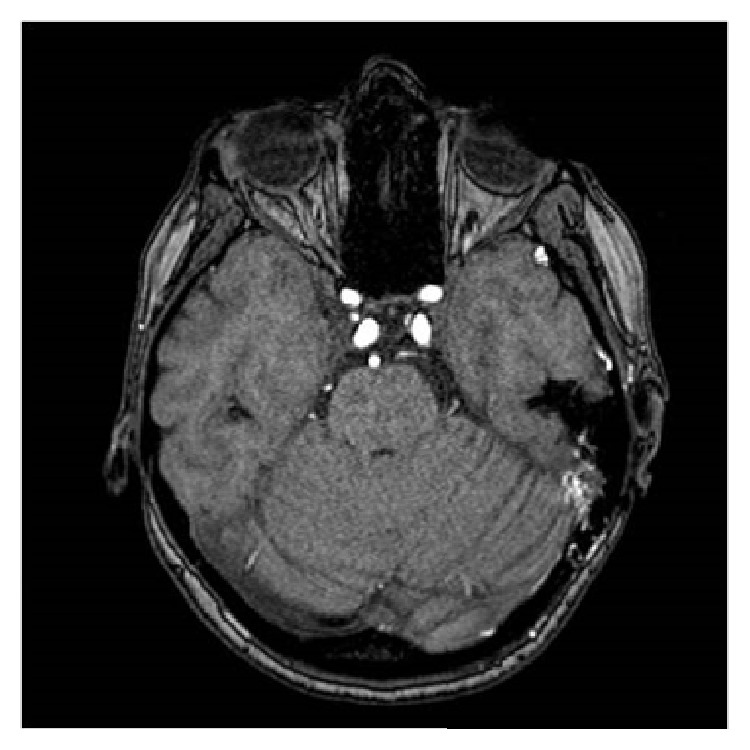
MRI of the brain: the vascular malformation, in the left occipitotemporal region.
